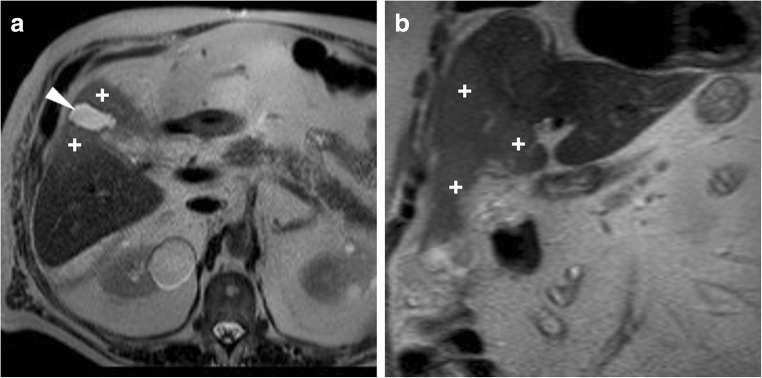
Expected MR appearance 24 h after uncomplicated laparoscopic cholecystectomy: axial (a) and coronal (b) T2-weighted images showing small-sized fluid collection in the gallbladder fossa, surrounded by increased signal intensity of the liver parenchyma (+), reflecting transient postoperative hepatic oedema
